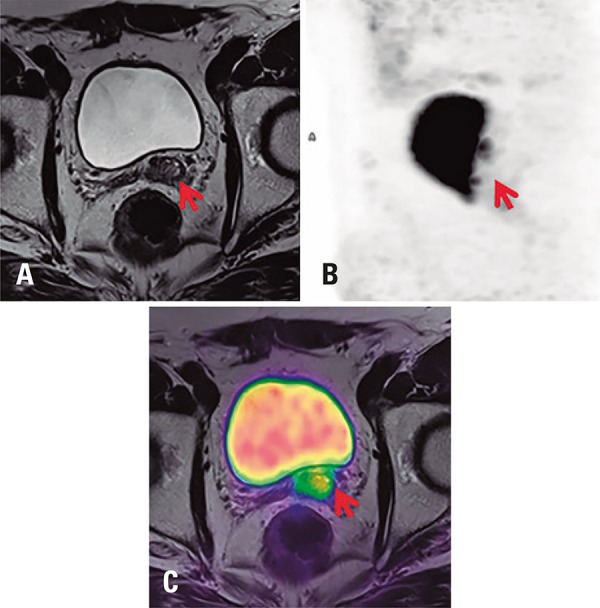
68Ga-PSMA PET/MRI image of a pelvis with recurrent prostate carcinoma in left seminal vesicle remnant, and marked PSMA expression in the lesion. (A) MRI image, axial T2; (B) PET, maximum intensity projection image; (C) fused PET/MRI image, axial T2
